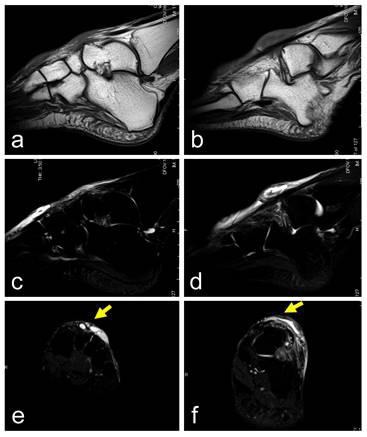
A 60-year-old female with a ganglion in the dorsal foot. The cystic lesions, with low signal intensity on a T1-weighted image (a–b) and high signal intensity on T2-weighted images (c–f), are located over the cuneiform to metatarsal bones (a, c, e) and the talus (b, d, f). The dorsalis pedis artery is located under the lesion (yellow arrows; e, f). T2-weighted images with fat-suppression (c-f). Sagittal views (a-d) and axial views (e–f).
